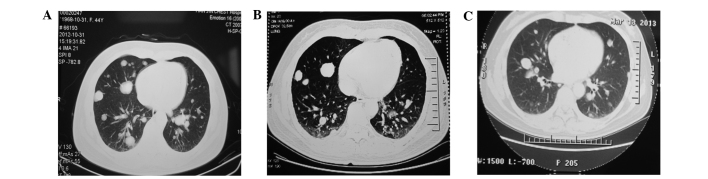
Chest computed tomography (CT) findings from different examinations. (A) CT revealing multiple variable-sized bilateral pulmonary nodules and a soft-tissue mass in the basal segment of the left-upper and bilateral lower lobes on Oct 31, 2012. (B) CT revealing that the multiple variable-sized bilateral pulmonary nodules were not reduced in size subsequent to therapy. The image was obtained on Nov 12, 2012. (C) CT revealing that the multiple variable-sized bilateral pulmonary nodules and lumps were increased in size on Mar 13, 2013.
